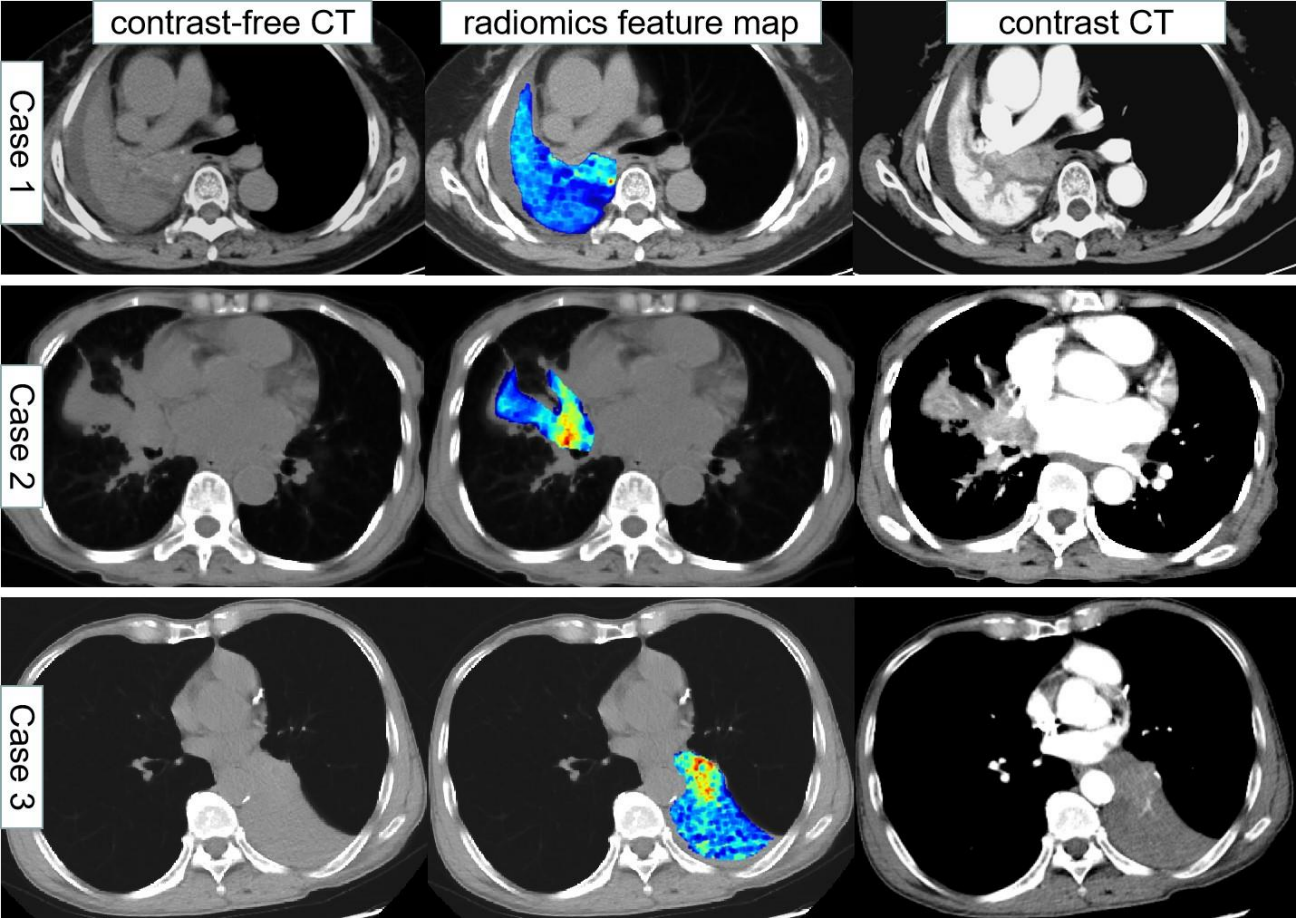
Visualization of radiomic features square_firstorder_10Percentile and comparison with contrast-free CT and contrast-enhanced CT images for three representative patients. The input images are axial slices of contrast-free CT scans focused on the lesion's largest cross-sectional area. Radiomic feature values for square_firstorder_10Percentile were calculated at the pixel level to generate radiomic feature maps, which were compared with the corresponding contrast-enhanced CT images. The radiomic feature maps reveal the distinct spatial distribution of square_firstorder_10Percentile, highlighting areas with significant differences between tumor and atelectasis.
